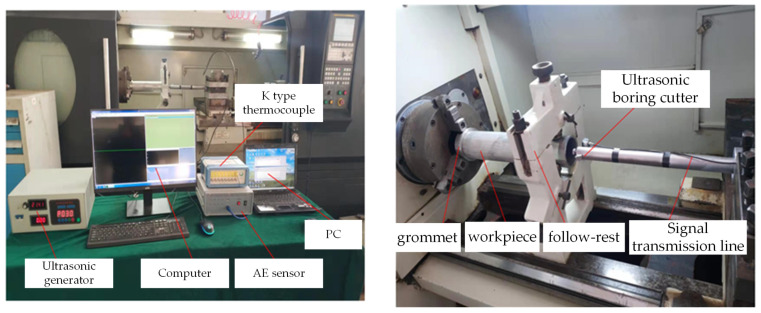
Two-dimensional ultrasonic vibration turning test platform.
